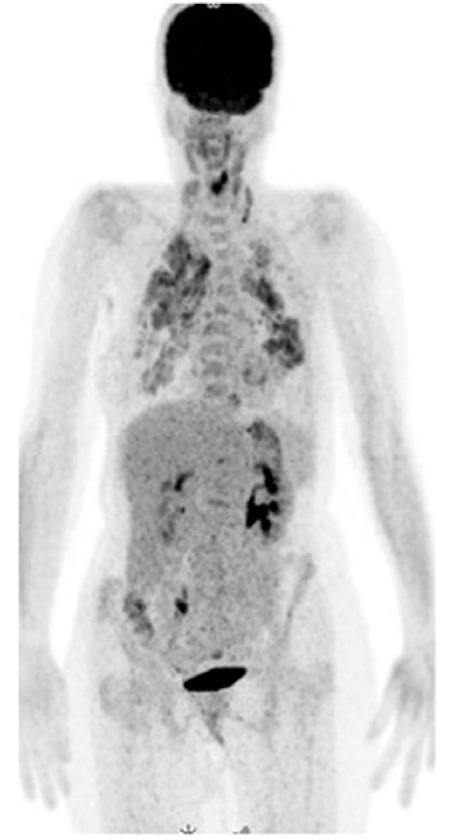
Whole body ^18^F-FDG PET/CT imaging revealed high FDG accumulation in the larynx, lung parenchyma and milimetric cervical lymph nodes
